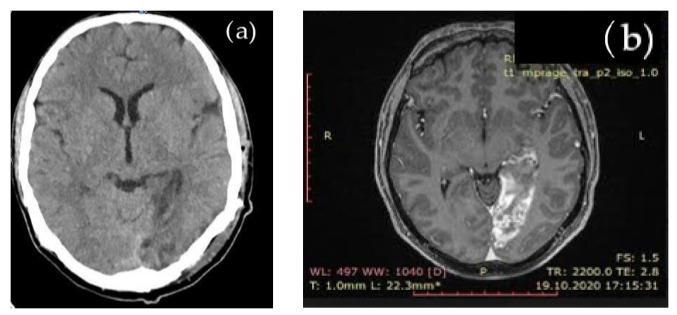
Cerebral imaging: (a) native brain CT aspect: left occipital ischemic hypodense lesion; (b) brain MRI aspect: left occipital ischemia.
